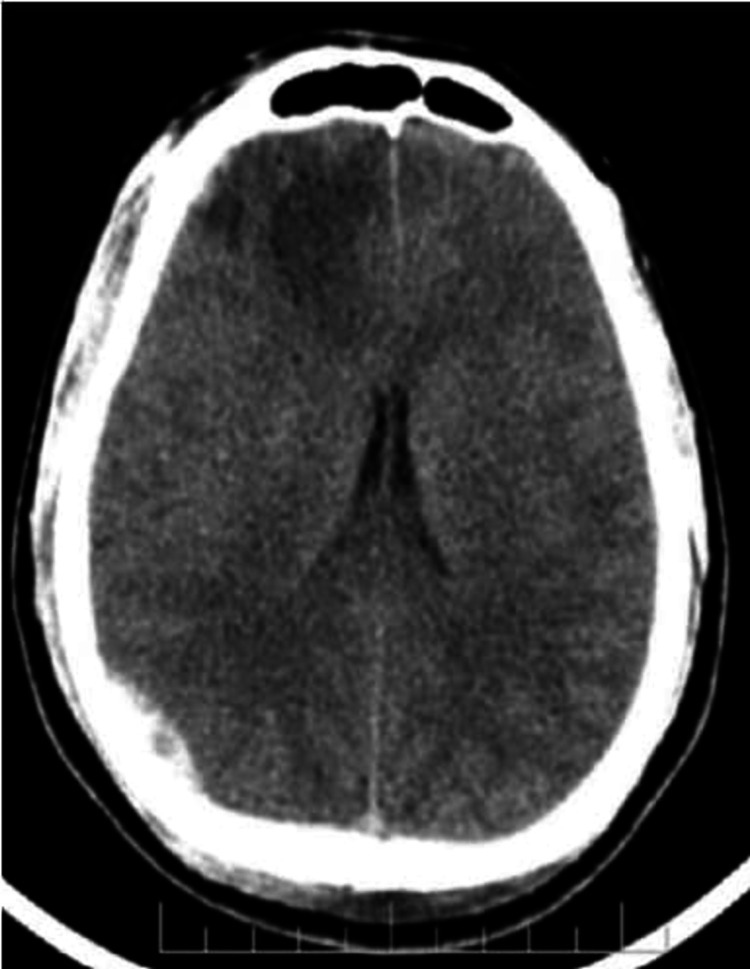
Axial NECT shows an intraparenchymal contusion in right frontal region, EDH in right parietal region with minimal mass effect compressing basal cisterns, no midline shift, subarachnoid hemorrhage noted - Rotterdam score 4 NECT: non-contrast-enhanced computed tomography; EDH: extradural hemorrhage
